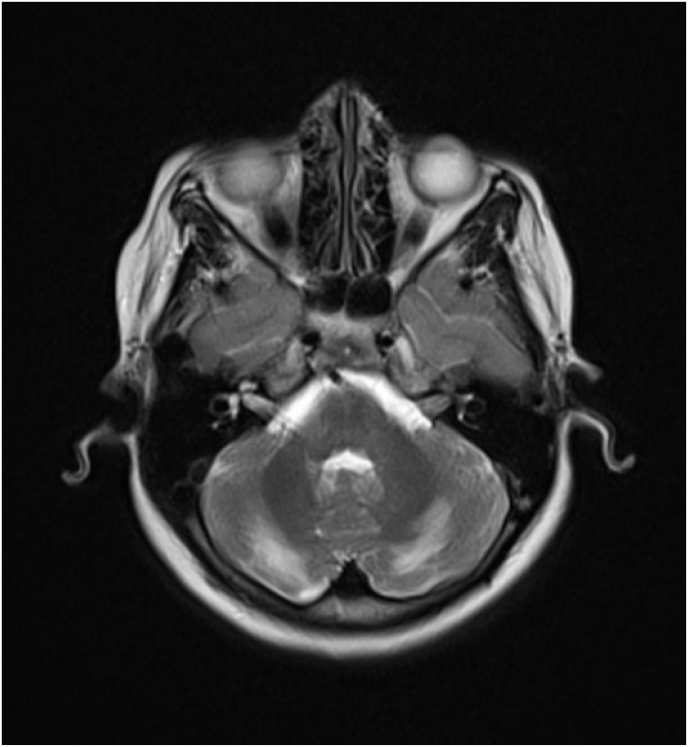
T2-weighted MRI showing high-signal subcortical band in the cerebellar hemispheres.
